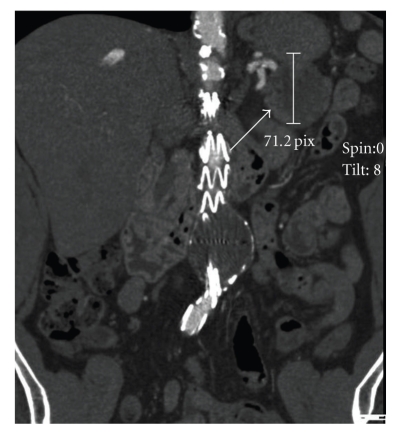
Coronal CT scan showing the tumor (arrow).
